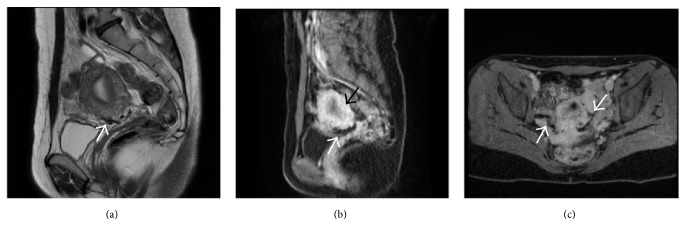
Postoperative MR 30 days after the embolization. (a) T2-weighted MR in sagittal plane: uterine wall preservation with serpiginous Onyx-18 cast (white arrow) into the left uterine artery. ((b) and (c)) Contrast-enhanced T1-weighted MR, sagittal and axial planes, respectively: preserved uterine wall perfusion (black arrow in (b)) without enhancement of the uterine arteries because of the Onyx-18 embolization (white arrows in (b) and (c)).
